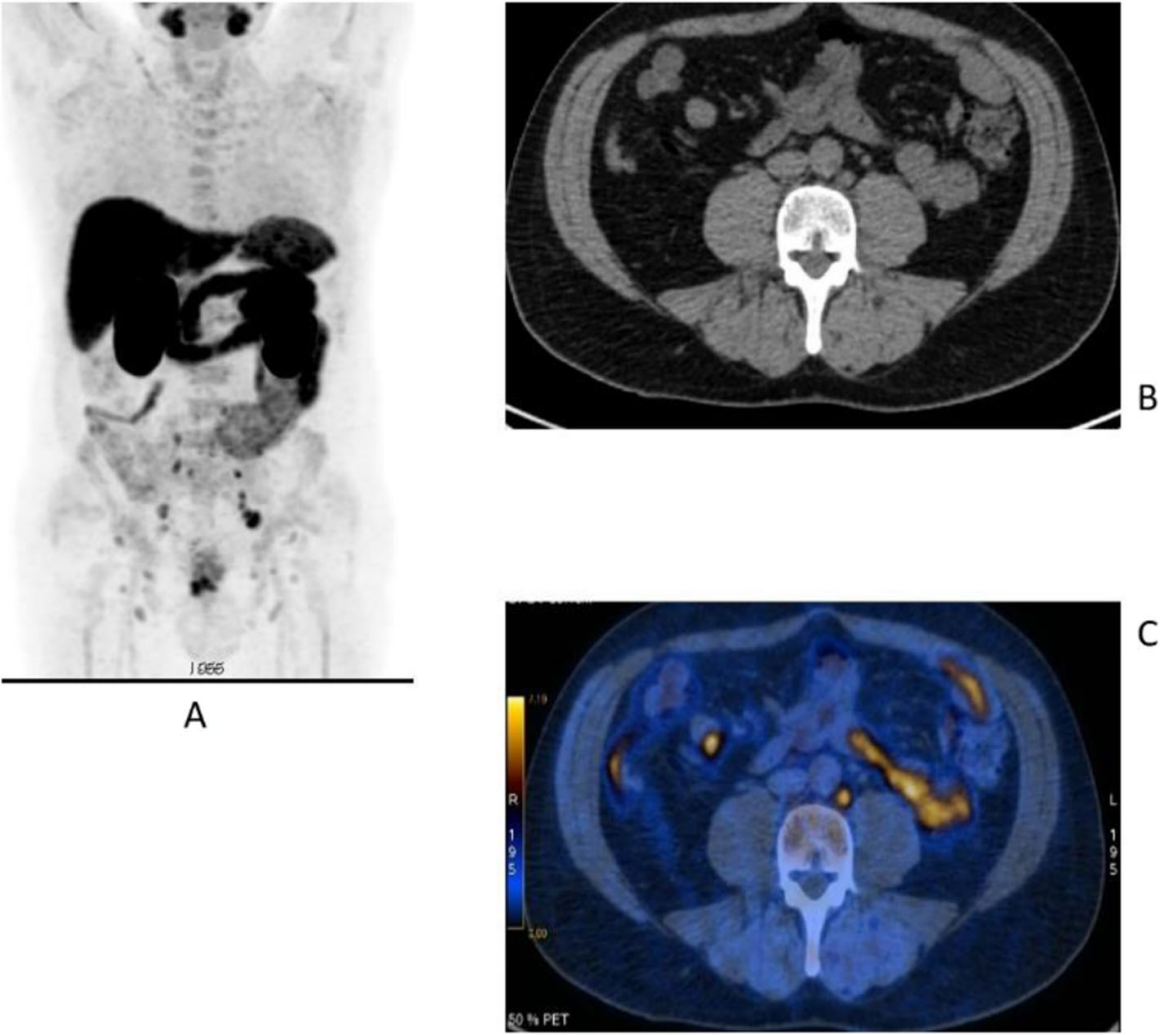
^11^C-choline, Initial staging: in very high-risk prostate cancer. *Clinical history*: 74 y.o. man with prostate cancer, Gleason score 4 + 5 according to biopsy. PSA = 126 ng/ml, T3a according to TRUS, candidate for radical prostatectomy. *PET/CT findings*: multiple foci of increased uptake seen through the pelvis and retroperitoneum, representing pathological uptake in the prostate and lymph node metastases. However, there is no evidence of osseous or visceral involvement. MIP (a), CT (b), and fused images (c)
